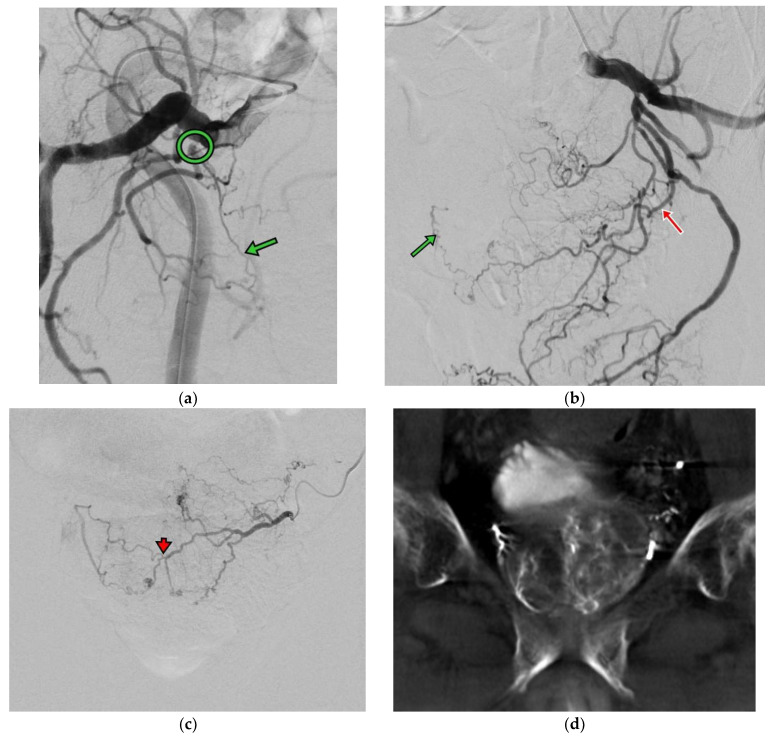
(a) Right internal iliac angiography (oblique view RAO 30 degrees) shows high grade stenosis (circle) at the branch of the right prostate artery (green arrow). (b) Left internal iliac angiography (oblique view LAO 30 degrees) shows the left prostate artery (red arrow) with contrast opacified at the right side of the prostate artery (green arrow). (c) Left prostate angiography (AP view) shows both sides’ prostate perfusion via intraprostatic anastomosis (arrowhead). (d) CBCT images show prostate perfusion at both sides on the left prostate angiography.
